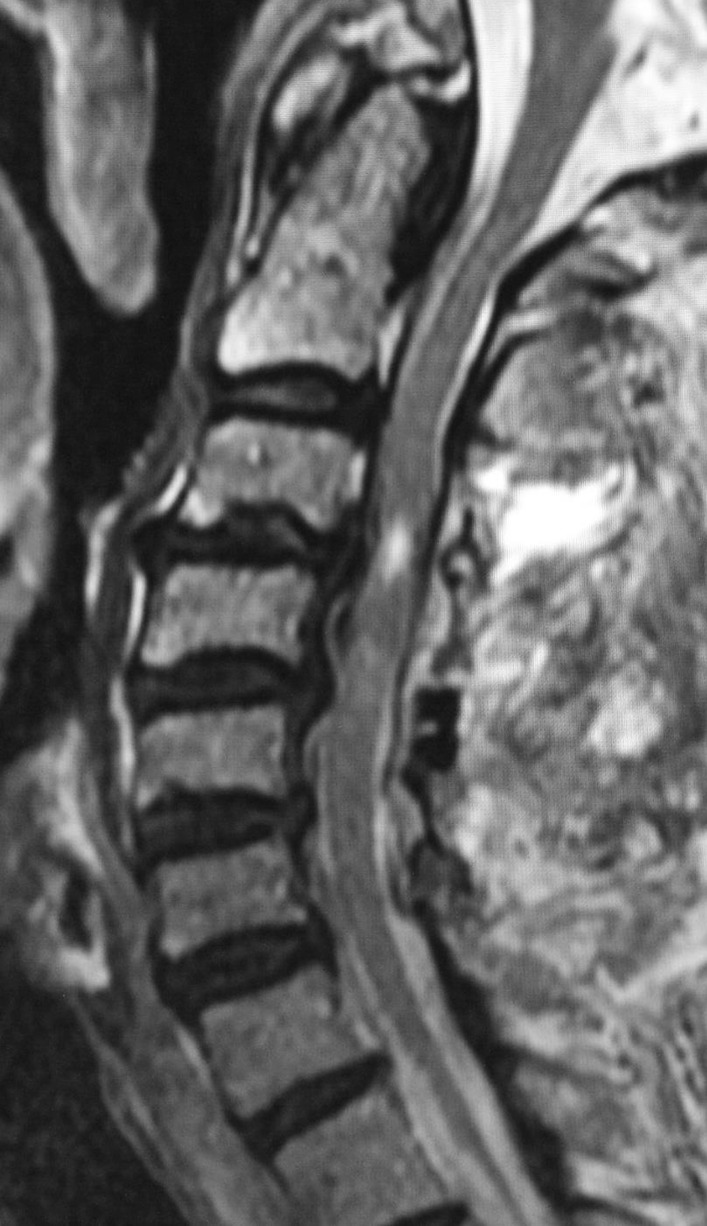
Postoperative magnetic resonance imaging (MRI) confirming successful decompression of the spinal cord
